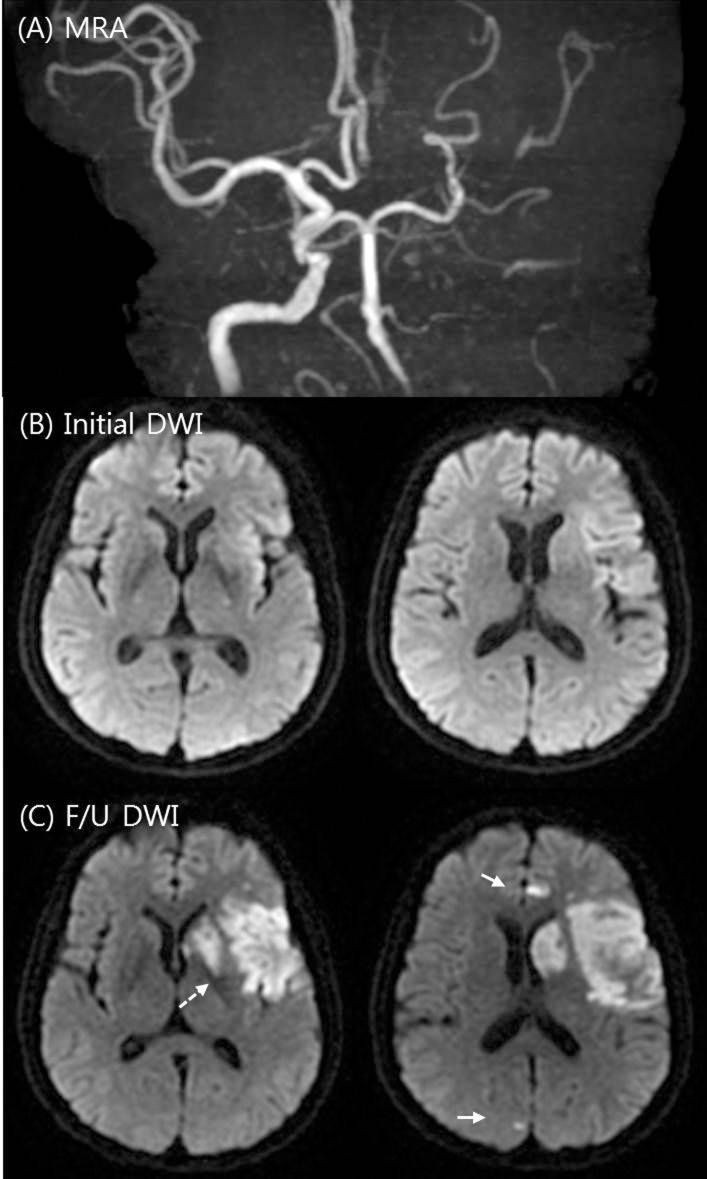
Example of a high percent insular ribbon infarction (PIRI) score. A 64-year-old man with an initial NIHSS score of 3 imaged 240 minutes after stroke onset. MRI showed left internal carotid artery occlusion on time-of-flight intracranial MRA (A), with subtle ischemic lesions with greater than 50% insular involvement on initial DWI (B; PIRI score 3). On the follow-up DWI (day 5), progressive insular infarction (dotted white arrow) with edematous changes and new ischemic lesions (white arrows) in the ACA and PCA territory were observed (C). Despite antiplatelet management, END occurred with an NIHSS score of 9.
